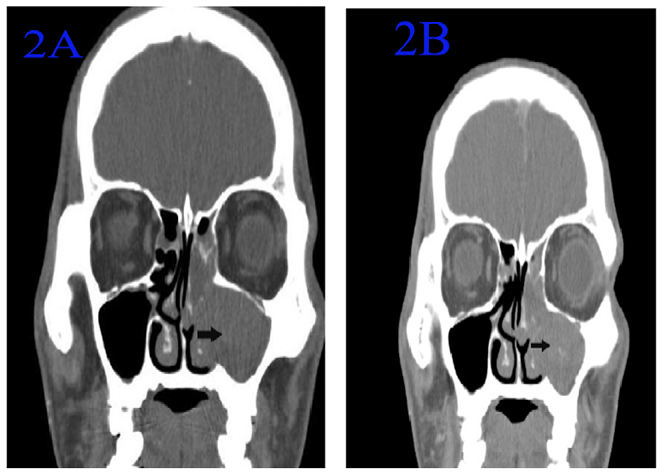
CT of an ANP in the left maxillary sinus involving the ipsilateral ethmoid sinus. 2A: Noncontrast coronal CT in soft-tissue window showed that a high-density mass filled the total left maxillary sinus. The density of the mass was homogeneous. The left maxillary sinus was expansile, and the medial maxillary sinus wall was partially destroyed (arrow). The mass involved the ipsilateral ethmoid sinus. 2B: Contrast-enhanced CT: The mass was non-enhanced.
